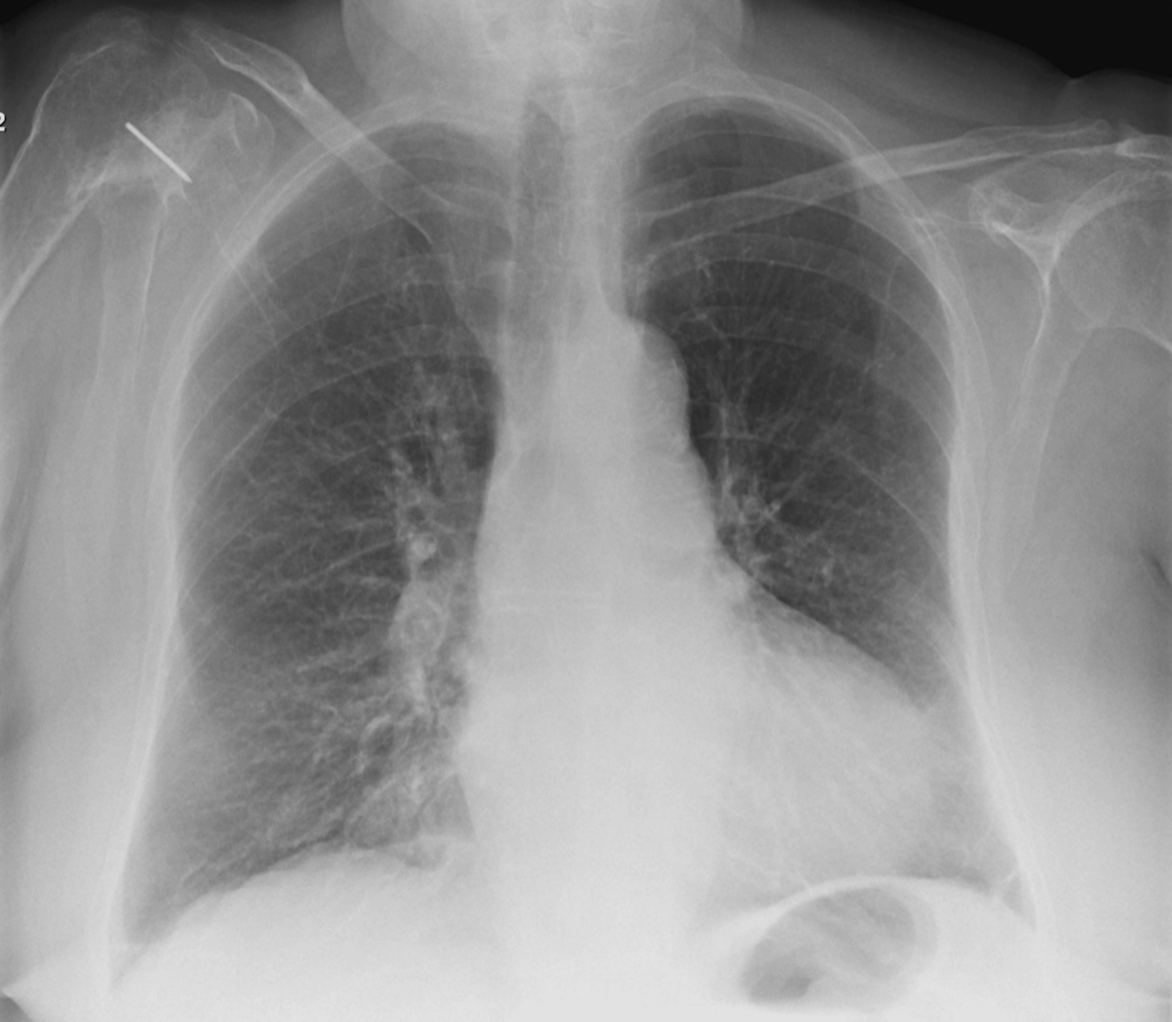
Chest X-ray three months prior to admission.
